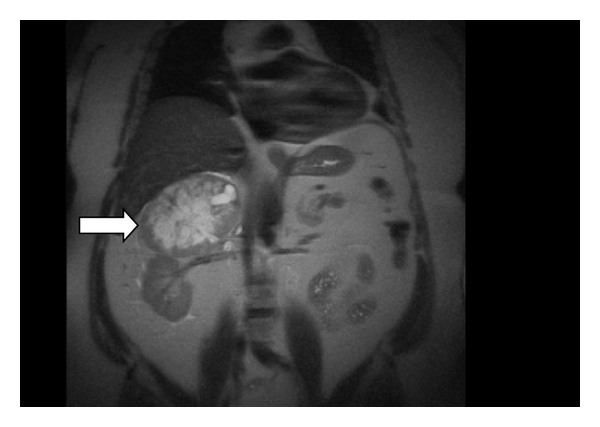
T2-Weighted MRI of the abdomen demonstrating high-attenuation characteristic of pheochromocytoma.
